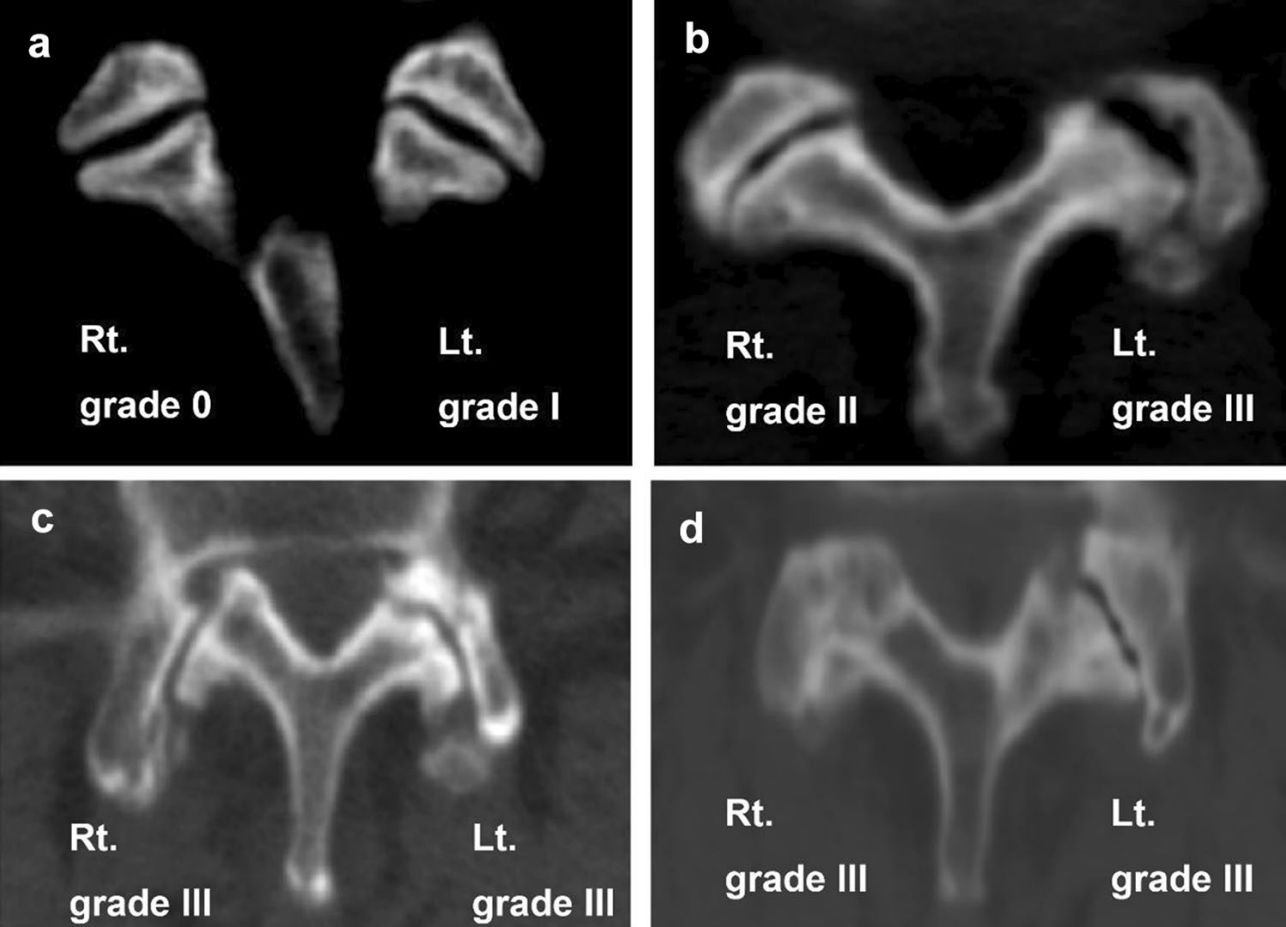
Representative CT images for 4 grades of facet joint arthropathy.
